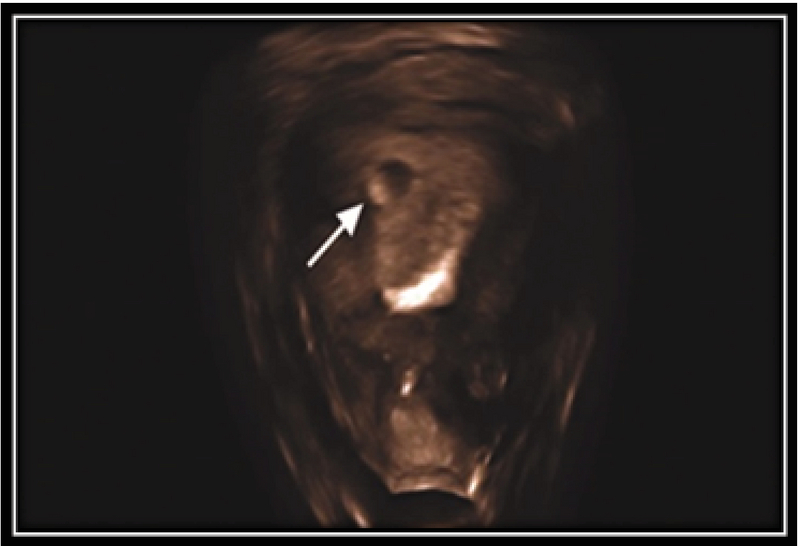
Intrauterine pregnancy: three-dimensional sonogram of the uterus shows the eccentric sac located within the endometrium (arrow).
